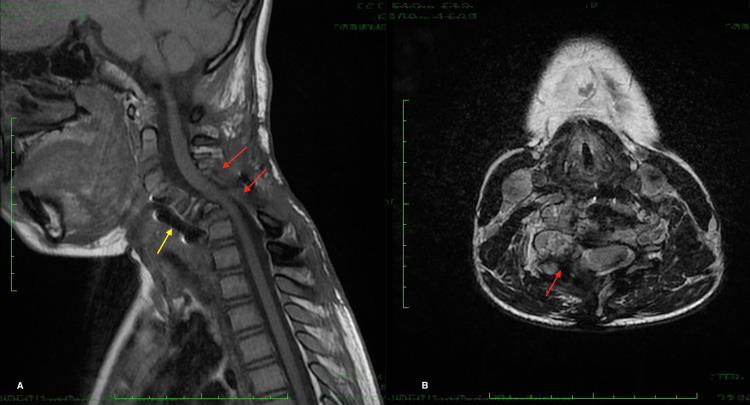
Sagittal (A) and axial (B) postoperative MRI showing posterior decompression (red arrow) with cervical kyphosis (yellow arrow)
